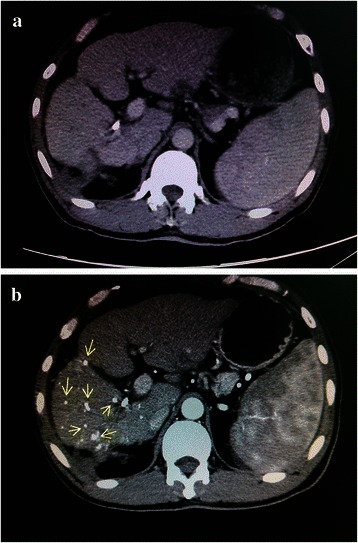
Multiple tiny lesions detected by lipiodol CT. a, no cancerous lesions were detected in contrast-enhanced CT prior to TACE. b, multiple tiny lesions with lipiodol depositions (as shown in *arrows*) were detected by using lipiodol CT after TACE in the same patient
